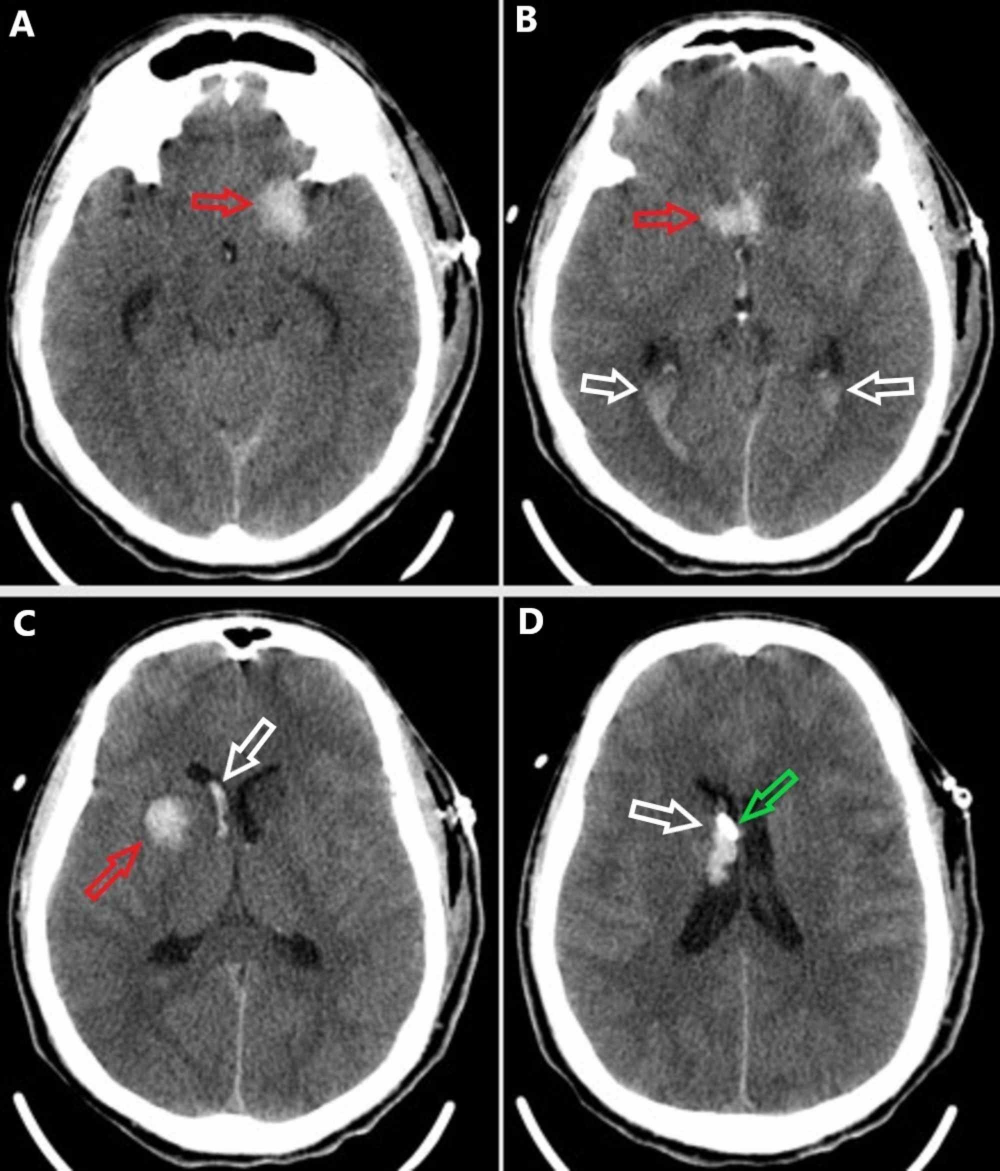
Postoperative noncontrasted head CT scan in the axial plane progressing from inferior (A) to superior (D). (A) There is an intraparenchymal hemorrhage in the left anterior temporal lobe along the injury tract (red arrow). (B) The intraventricular blood is seen layering into the right occipital horn of the lateral ventricle and the bilateral atria (white arrows). Injury tract hemorrhage is seen in the midline (red arrow). (C) There is a tract hemorrhage within the right basal ganglia (red arrow) and intraventricular hemorrhage within the right lateral ventricle frontal horn and body (white arrow). (D) The external ventricular drain tip (green arrow) is visible adjacent to the right lateral intraventricular hemorrhage (white arrow)
